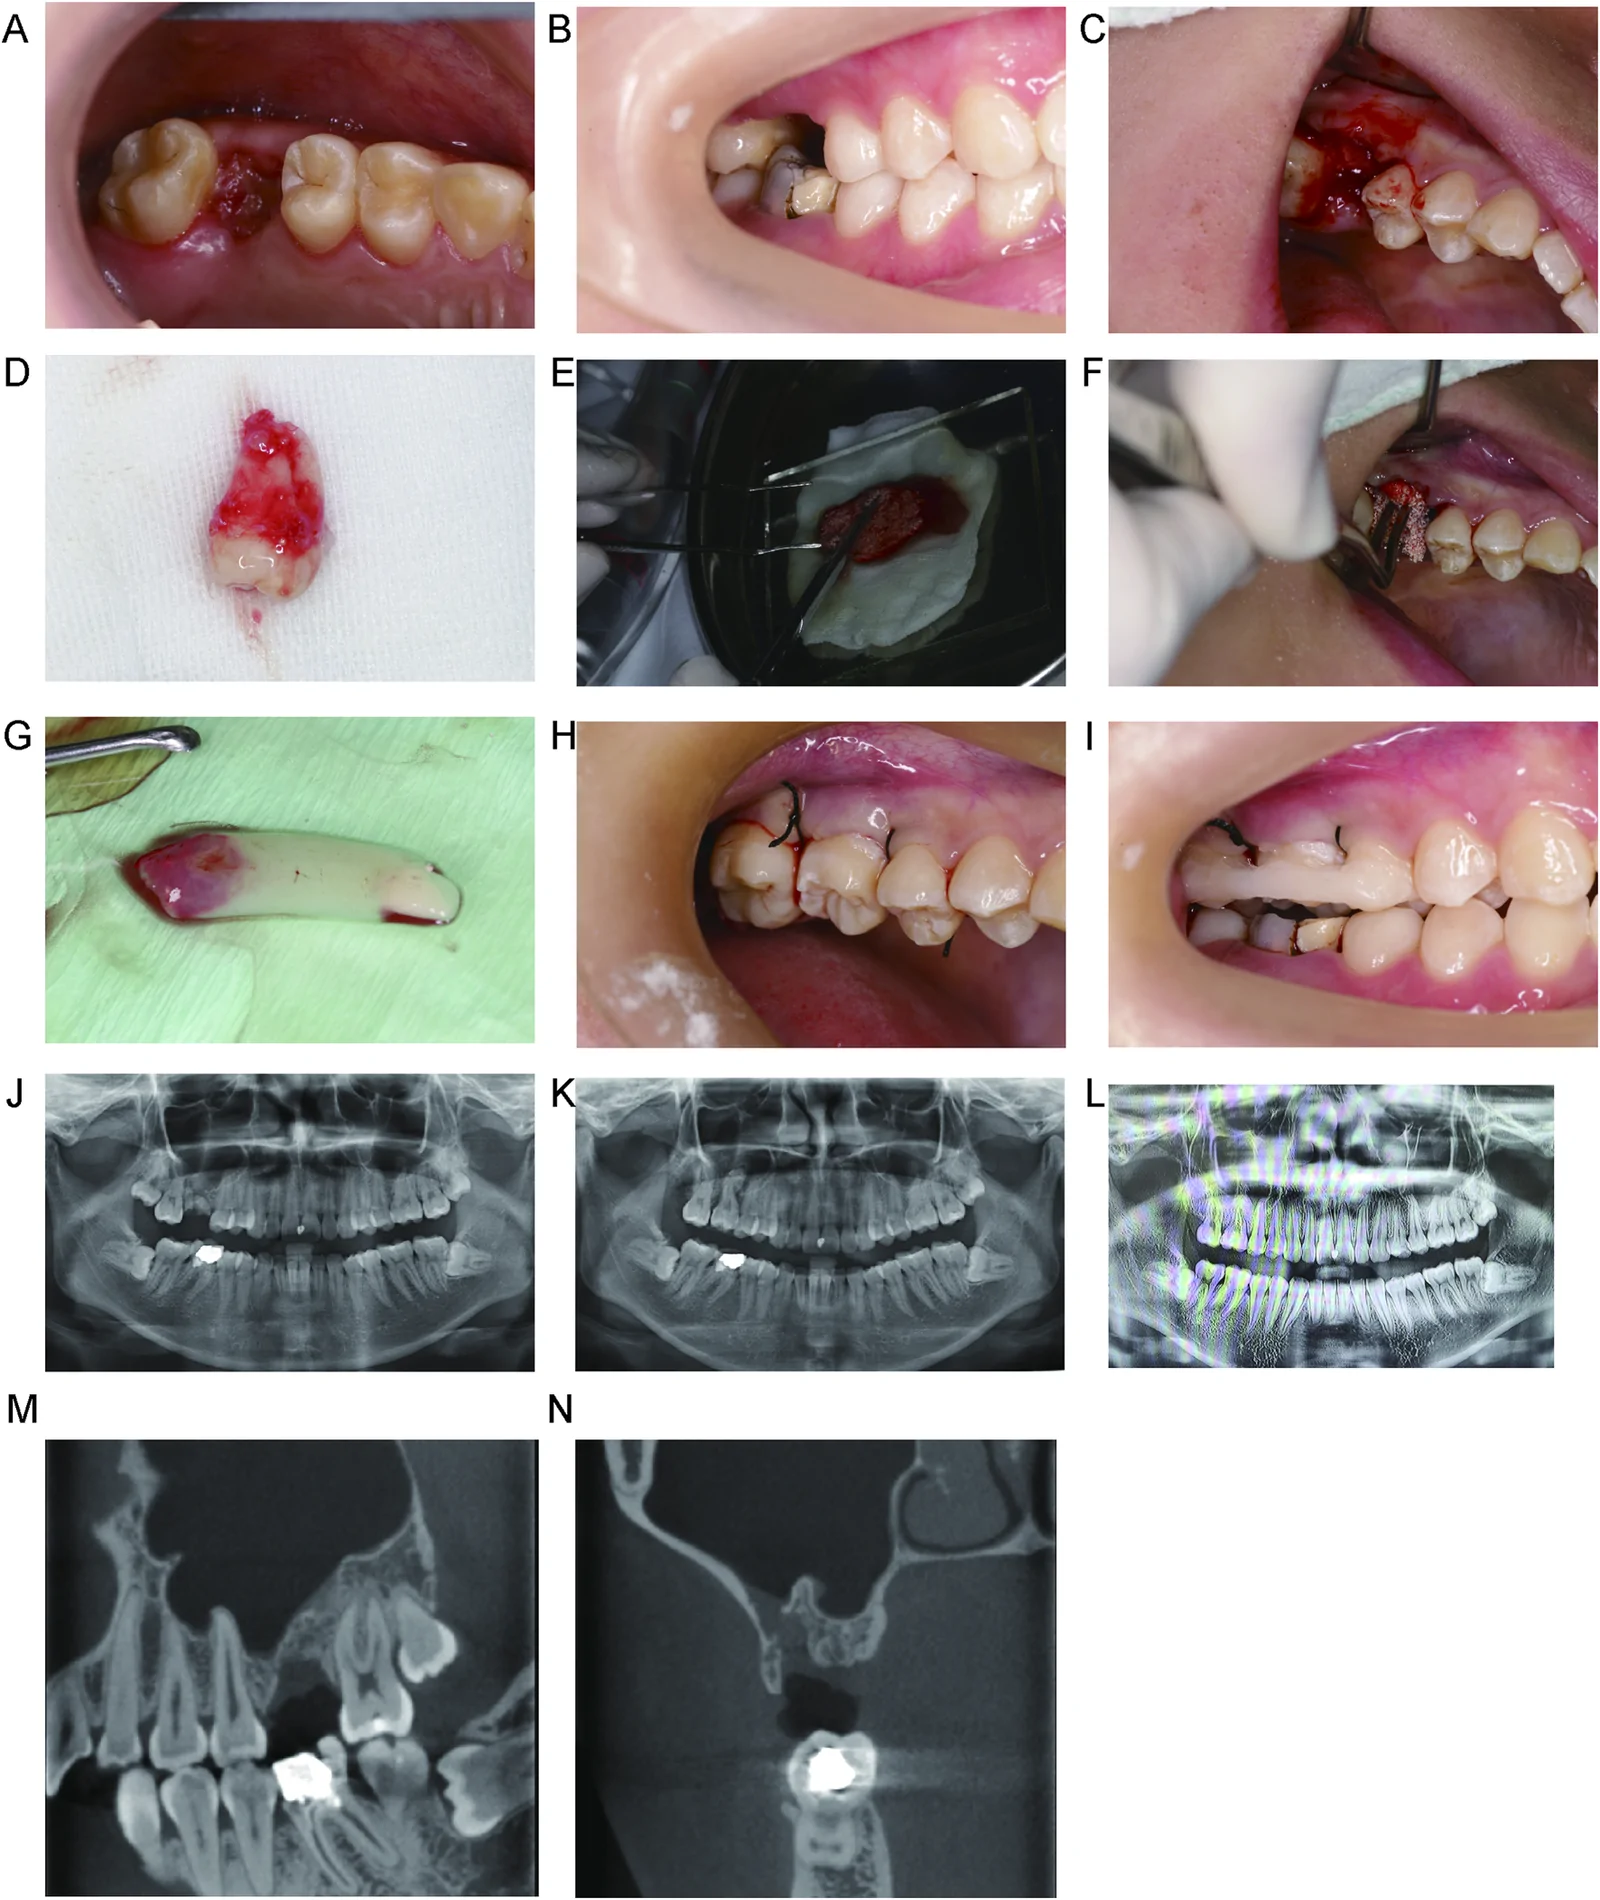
Clinical photographs and radiographic images of Case 3 showing autogenous tooth transplantation of tooth 18 to the tooth 16 recipient site with sinus-floor defect management. (A,B) Initial intraoral photographs of tooth 16. (C) Minimally traumatic extraction of tooth 16. (D) Minimally traumatic extraction of donor tooth 18. (E) Preparation of sticky bone. (F) Placement of sticky bone into the alveolar socket defect at the tooth 16 recipient site. (G) Preparation of concentrated growth factor. (H and I) Stabilization of transplanted tooth 18 at the tooth 16 recipient site. (J) Preoperative panoramic radiograph before autogenous tooth transplantation. (K) Immediate postoperative panoramic radiograph after transplantation. (L) Panoramic radiograph at the 1-year follow-up. (M,N) Preoperative CBCT before autogenous tooth transplantation. ATT, autogenous tooth transplantation; CGF, concentrated growth factor.
